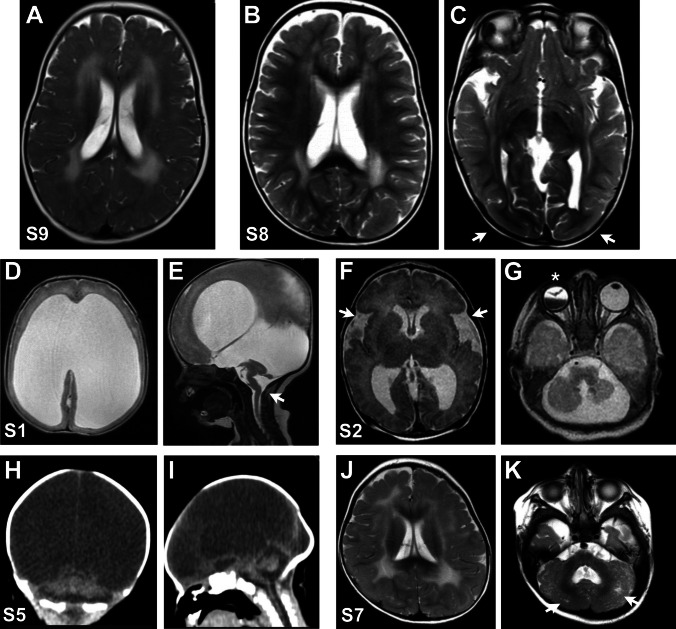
Brain imaging for selected cases. A Axial T2 MRI image showing the classical T2 hyperintense signal within the periventricular white matter in case S9. B, C Axial T2 MRI images of case S8 showing abnormal hyperintense signal within the periventricular white matter with additional cortical malformations including bilateral occipital segmental lissencephaly (arrows in C). D, E: Axial (D) and sagittal (E) T2 MRI images of case S1 showing diffuse cobblestone type II lissencephaly with flattening of the entire cerebral mantle as well as hypoplastic cerebellum and pons and Z-shaped brainstem (arrow in E). F, G: Different axial T2 MRI images of case S2 showing supratentorial abnormal perisylvian cortical development (arrows in F), cerebellar hypoplasia, small size pons, and abnormal right eye globe with abnormal right lens configuration and intra vitreous hemorrhage (asterisk in G). H, I: Coronal (H) and sagittal (I) non contrast CT images of case S5 showing the supratentorial brain nearly replaced by CSF apart from the interhemispheric fissure, also associated with hypoplastic cerebellum and kinked brain stem structures. J, K: Axial T2 MRI images of case S7 showing periventricular white matter abnormalities in addition to hypoplasia of both cerebellar hemispheres with multiple bilateral microcysts as well as dysplastic features
